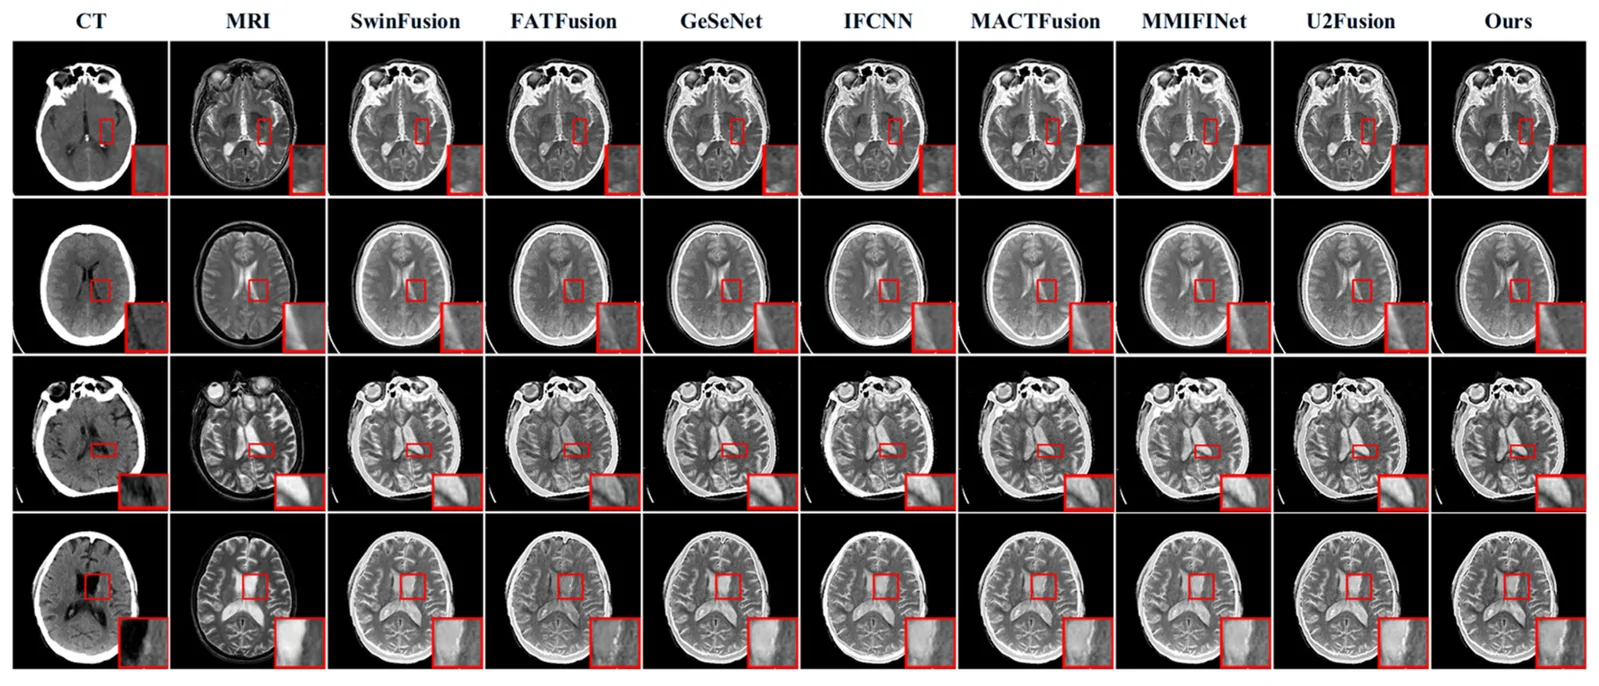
Qualitative comparison of fusion results on the CT–MRI dataset. The columns display the source CT, source MRI, and the fused results from seven state-of-the-art methods alongside our proposed MSFusion. Zoomed-in regions (highlighted by colored boxes) demonstrate that MSFusion preserves sharper anatomical boundaries and finer textural details (e.g., bone structures and soft tissue interfaces) with fewer artifacts compared to competing methods, corroborating its superior quantitative performance in MSE and PSNR.
